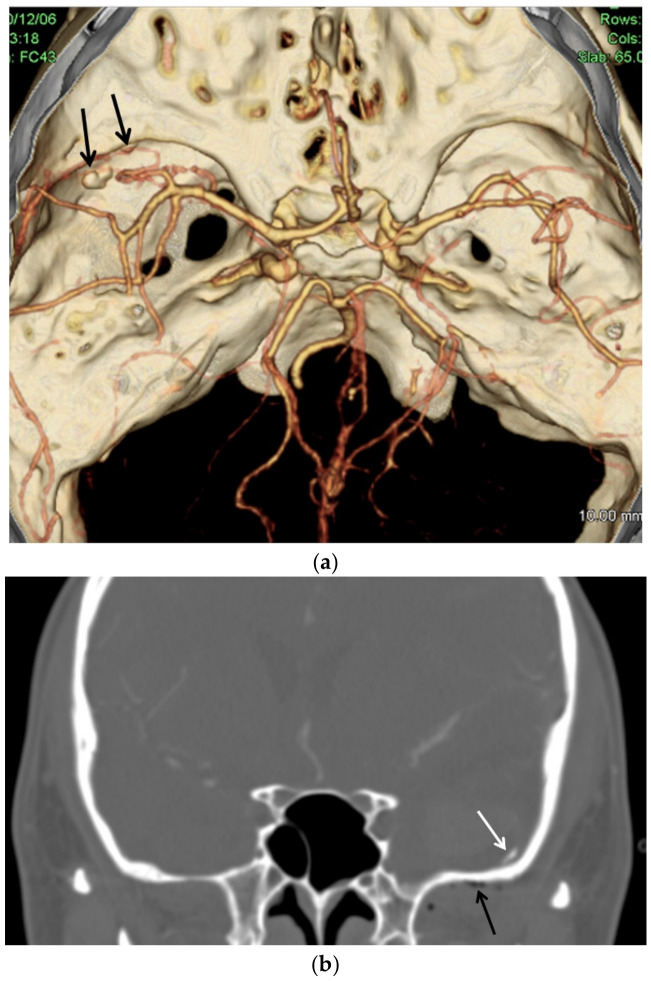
Brain computed tomography angiography (a) demonstrates bony abnormalities presumed to have been caused by improper use of a reciprocating saw during surgery (black arrows: bony abnormalities). Coronal computed tomography (b) shows a bony fragment (white arrow) within the intracranial cavity and an air bubble (black arrow) in the extracranial region of the left middle cranial fossa. (These images correspond to Figure 6 in Kim et al. [3]. Permission to reproduce the images was obtained from the Editor-in-Chief of the *Korean Journal of Neurotrauma*).
